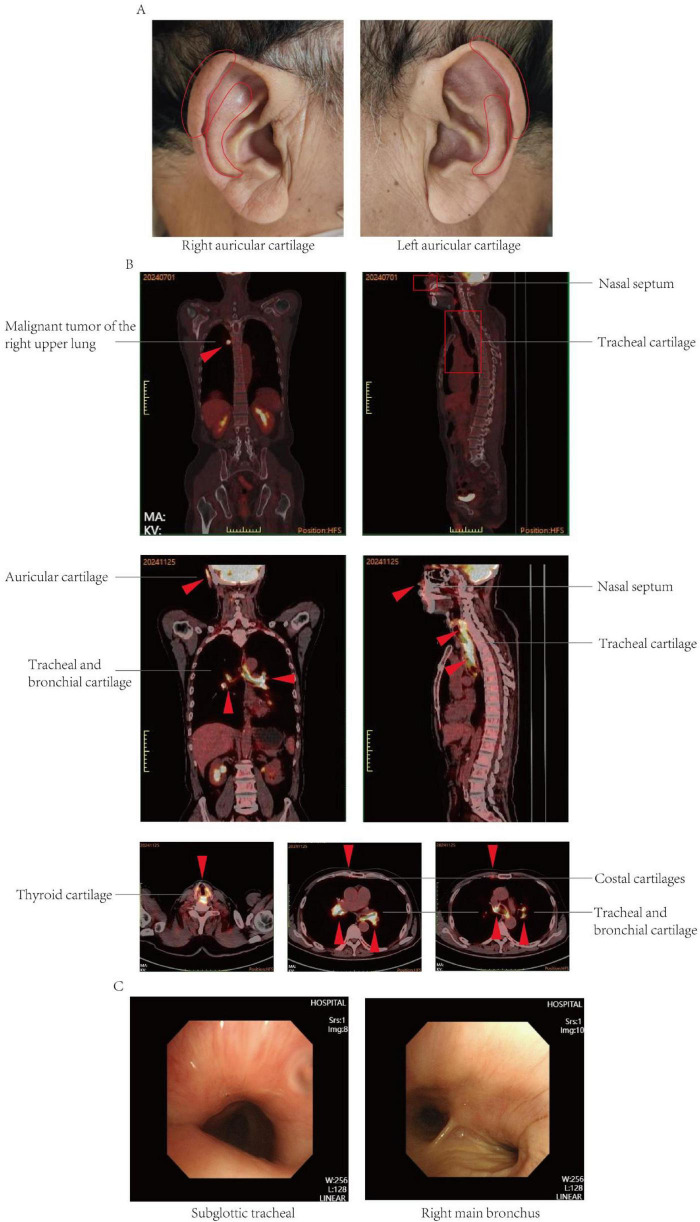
(A) Clinical pictures at presentation. Note the distinctive swelling and redness of the right auricular cartilage and left auricular cartilage. (B) 18F-FDG PETCT imaging. Preoperative 18F-FDG PET/CT imaging revealed a nodular lesion in the right upper lobe of the lung exhibiting increased metabolic activity, suggestive of a malignant tumor. The preoperative 18F-FDG PET/CT imaging did not demonstrate increased metabolic activity in the nasal septal and tracheal cartilage. Postoperative 18F-FDG PETCT showed that the density of cricoid cartilage was higher than before, and the walls of trachea and bronchus were abnormally thick and dense, and the nasal septum, bilateral auricular cartilage, bilateral thyroid cartilage, and bilateral costal cartilage were with diffuse abnormal FDG metabolism, which was considered relapsing polychondritis. (C) Bronchoscopy of tracheal stenosis in present case report. The procedure revealed a subglottic tracheal collapse accompanied by mucosal hyperplasia; the right main bronchus exhibited signs of softening and collapse.
